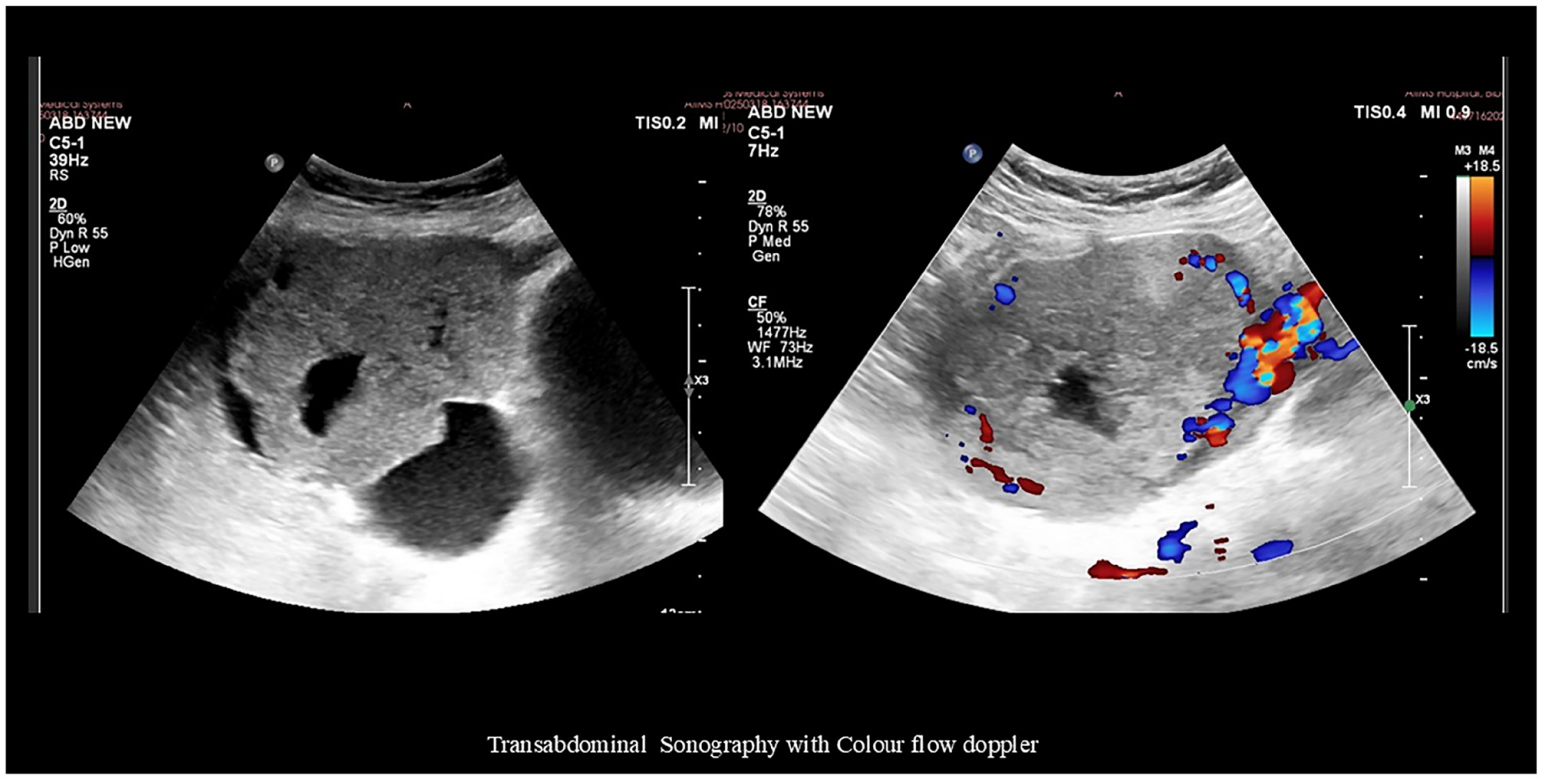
Transabdominal sonography image revealing a well-defined, predominantly solid-cystic lesion (10.2 × 7.8 × 7.8 cm) with vascularized solid components in the right adnexa with areas of hemorrhage.
